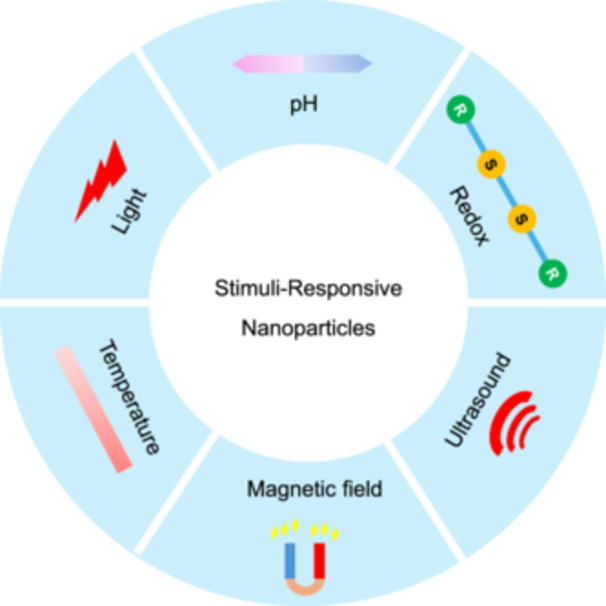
Factors for stimuli‐responsive release strategies. Factors influencing stimuli‐responsive release strategies include light, pH, redox potential, temperature, magnetic field, and ultrasound. These stimuli can be harnessed to trigger the controlled release of therapeutic agents from drug delivery systems, offering potential applications in targeted and responsive drug delivery. Modified from reference 27, https://doi.org/10.1186/s12951-022-01309-9.
